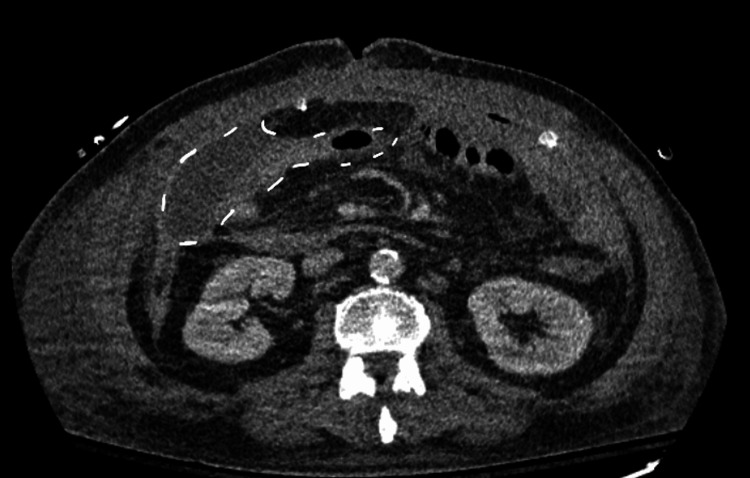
CT abdomen and pelvis with contrast - image 5 White dotted lines indicate remaining fluid collection. This shows improvement compared to Figure 4
